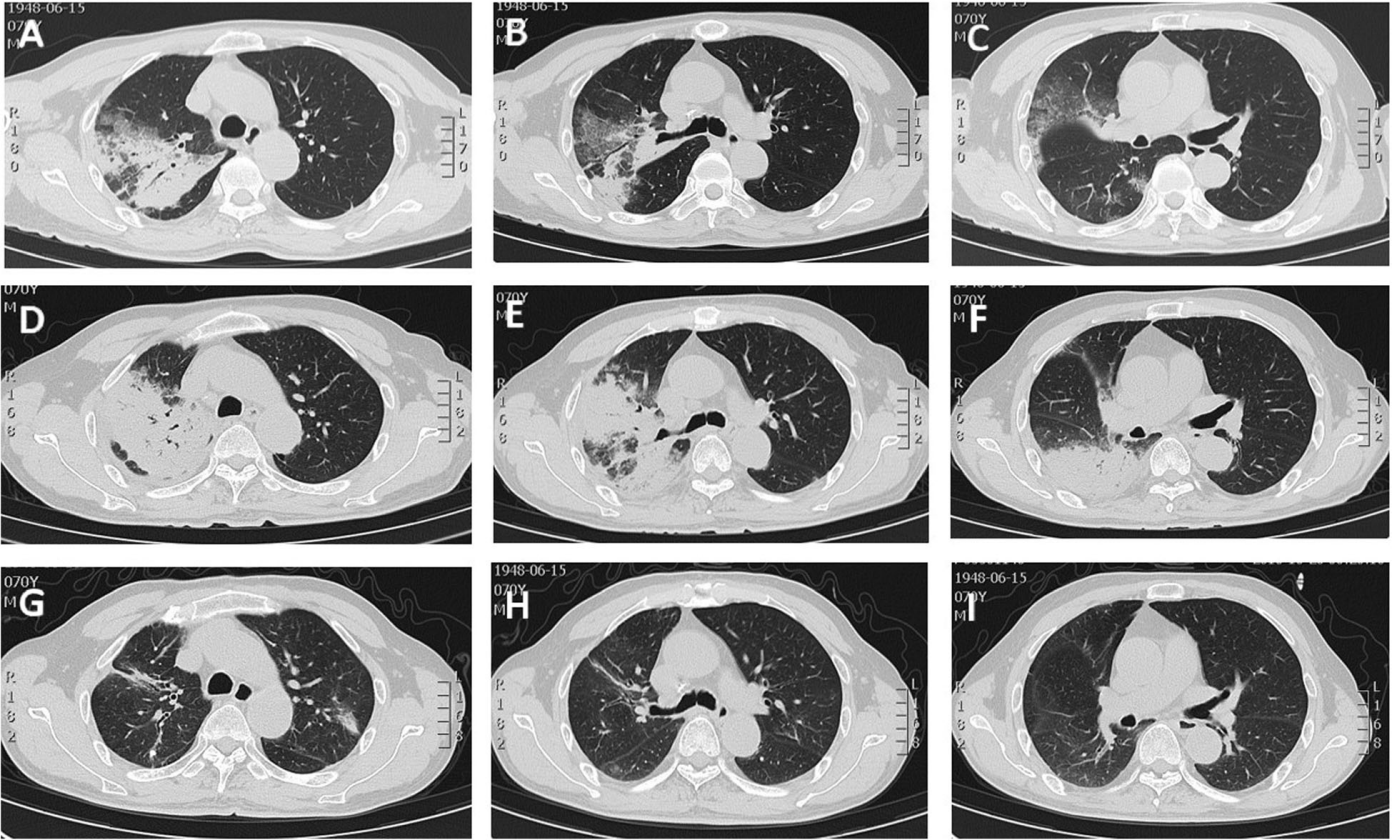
In case 1, CT scan (a, b and c) showed consolidation of the right upper lobe with an air bronchogram. Ten days after treatment with antibiotics, repeat CT scan (d, e and f) showed more extensive lesion than previously observed. After treatment with corticosteroids, CT scan (g, h and i) showed that the lesion was absorbed
